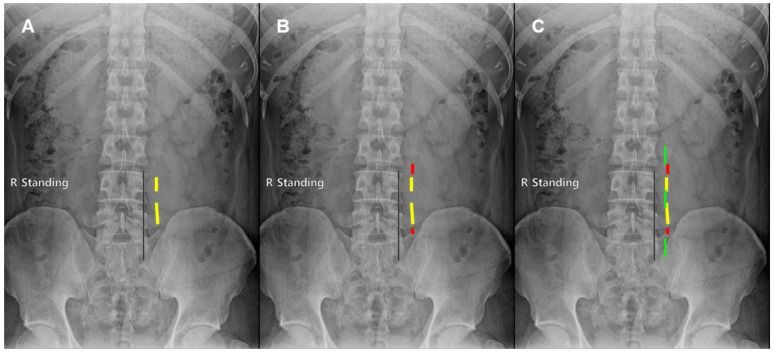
Stepwise procedure of biportal endoscopic foraminotomy and monosegment screw fixation on radiographic view. (A) Biportal endoscopic foraminotomy is performed with two small incisions (yellow lines). The black line indicates the pedicle lateral margin. (B) After nerve root decompression through foraminotomy, the incision is slightly extended to allow for percutaneous pedicle screw fixation (red lines). (C) After pedicle screw insertion, the incision is further extended and the fascia is incised to insert the dynamic rod (green lines).
